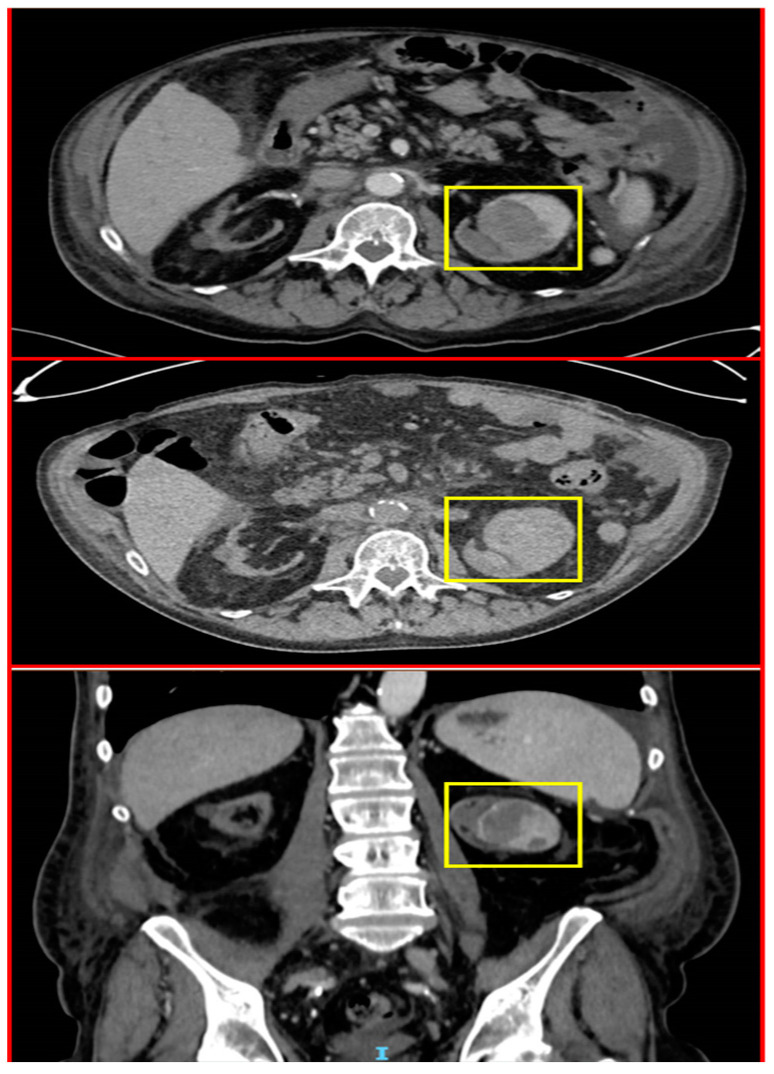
Images from the patient’s CT during investigation of hematuria showing a mass in the upper pole of the left kidney (yellow squares).
